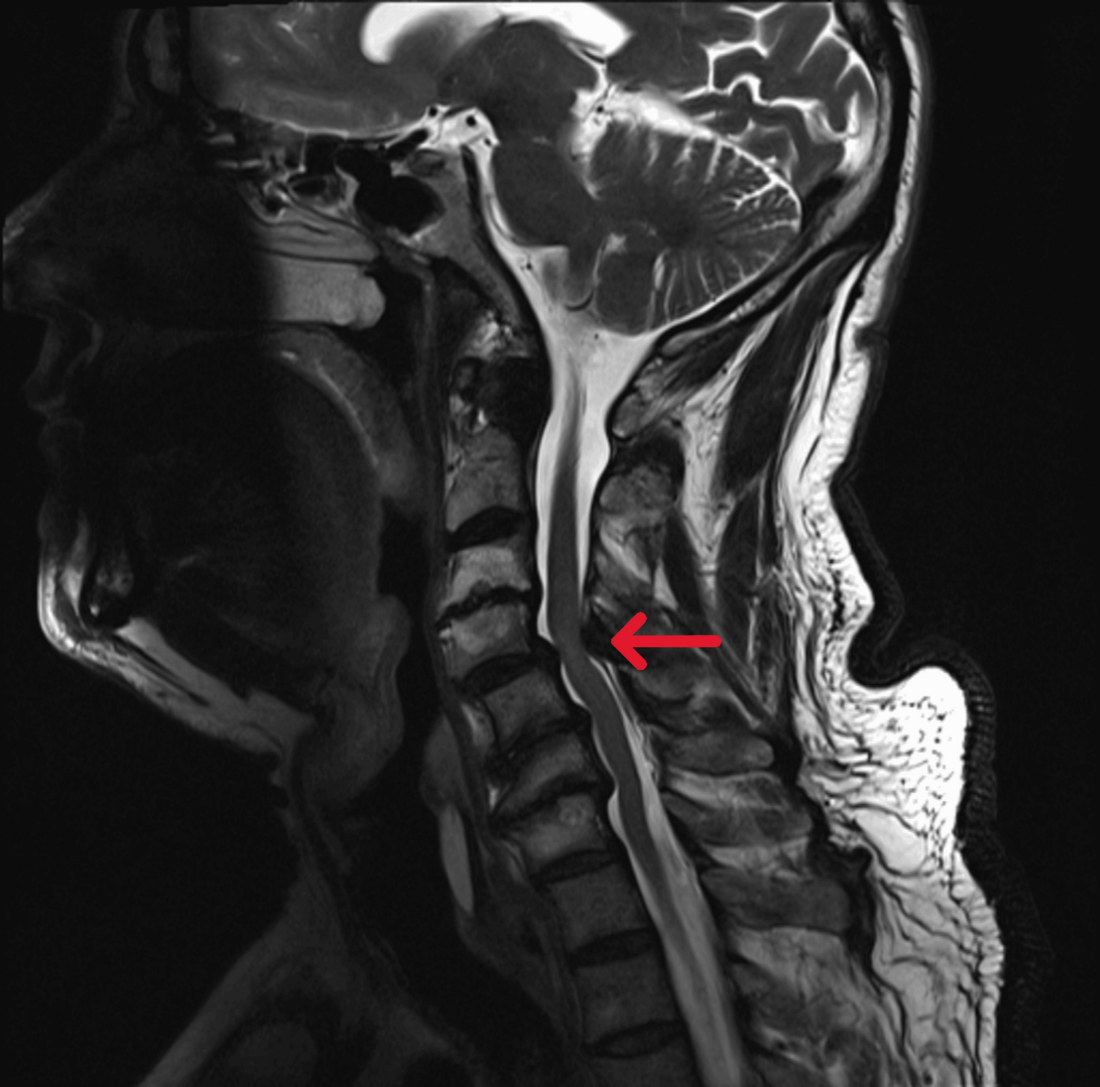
Cervical MRI Cervical MRI (T2-TSE, sagittal plane) showing reduced anteroposterior diameter of the spinal canal with indentation of the spinal cord at the C4-C5 level.
